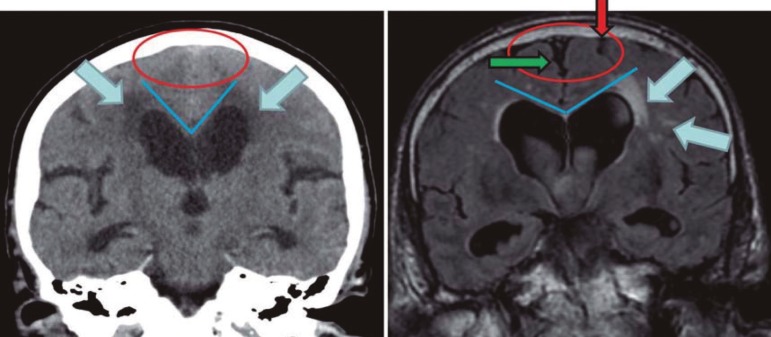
Coronal head CT (left) and MRI (right) at the level of the posterior
